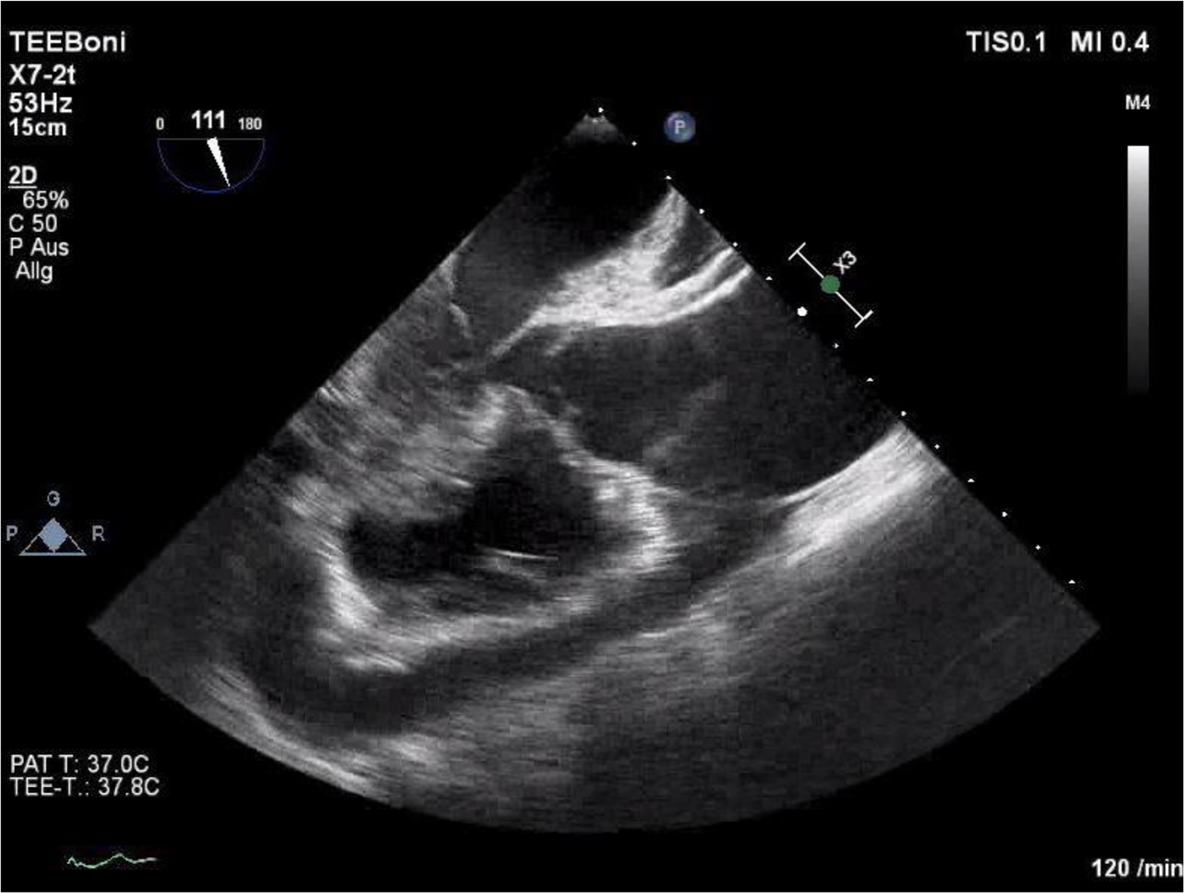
Transoesophageal echocardiography demonstrating pericardial effusion and dissection
